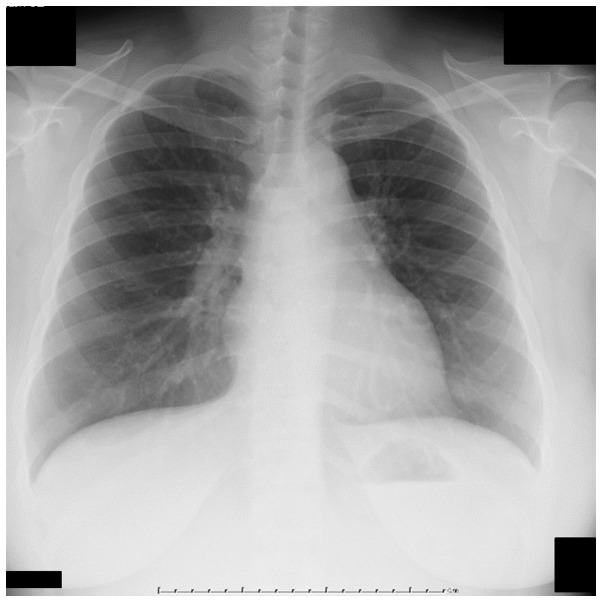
Chest roentgenogram showing no lung lesions or swollen hilar lymph nodes.
